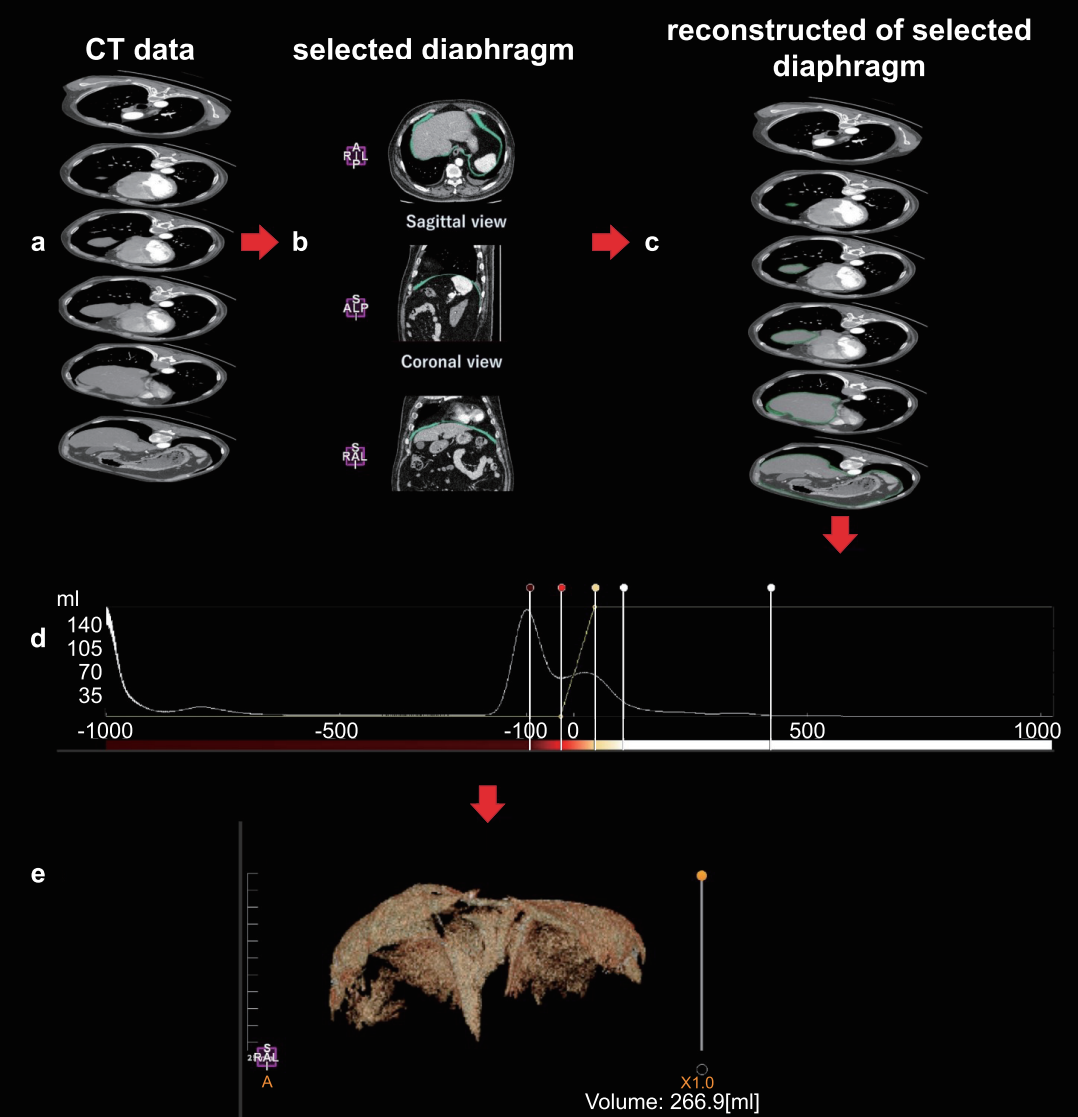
Operating steps for the assessment of the diaphragm volume during deep inspiration. (a) All computed tomography (CT) data are imported into the workstation software. (b) The diaphragm is selected and extracted. (c) The diaphragm is reconstructed. (d) The color mapping operation screen is shown. (e) The volume is measured.
